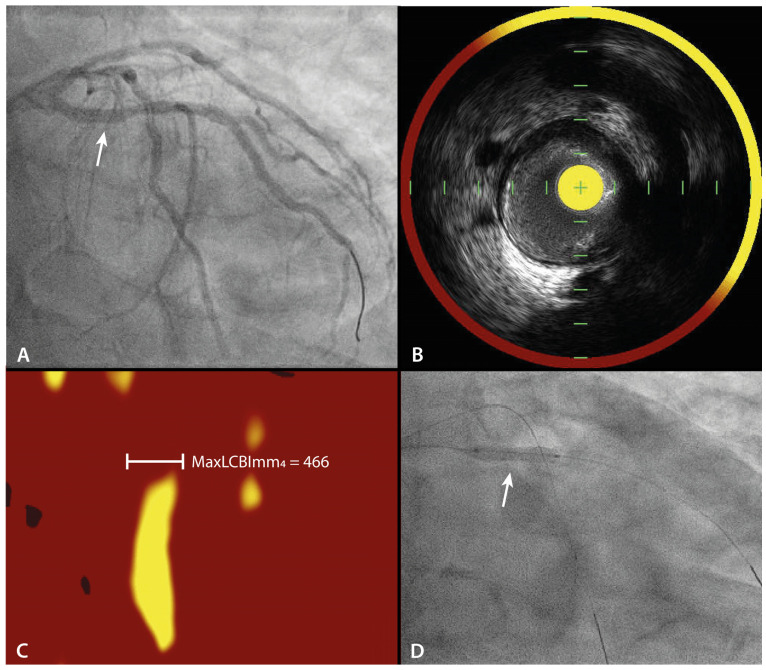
Case example. (A) Depicts the coronary angiogram, wherein the proximal circumflex artery, as highlighted with the white arrow, a lipid-rich plaque was observed with intravascular ultrasound (IVUS, (B)) and near-infrared spectroscopy (NIRS, (C)). (D) This lesion was subsequently treated with paclitaxel-eluting drug-coated balloon (PE-DCB) as highlighted with the white arrow.
